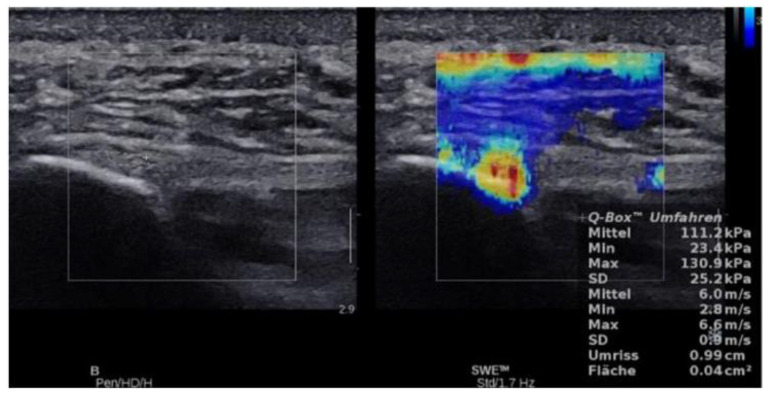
Longitudinal SWE image and B-mode ultrasound image of a 30 years old healthy volunteer with normal plantar fascia demonstrates stiffness of 111.2 kPa and SWV value of 6.0 m/s.
